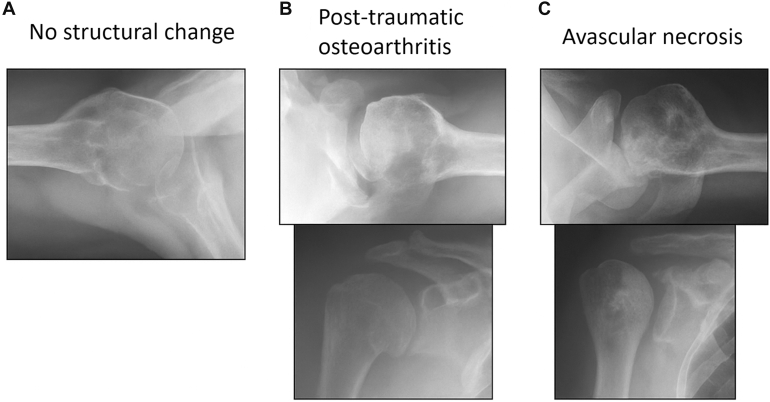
Representative radiographs showing typical long-term outcomes following locking plate fixation of proximal humerus fractures. (A) No structural change; (B) development of post-traumatic osteoarthritis with joint-space narrowing and osteophyte formation; (C) avascular necrosis with segmental collapse of the humeral head.
